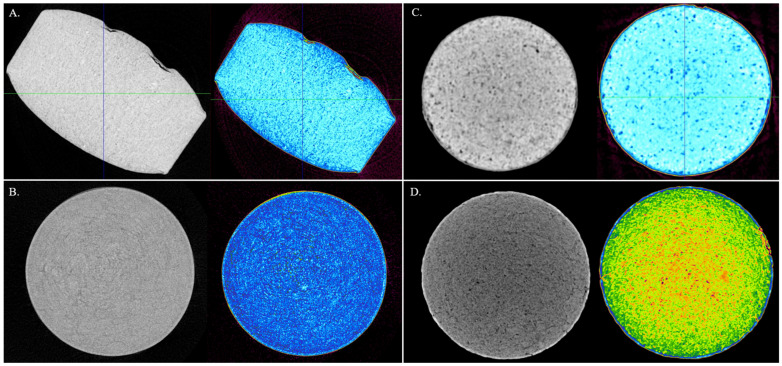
Images captured via X-ray Computed Tomography: (A) Tablet A; (B) Tablet B; (C) Tablet C; (D) Tablet D. Images are shown in grayscale and color-scale to visualize film coat and core interface.
